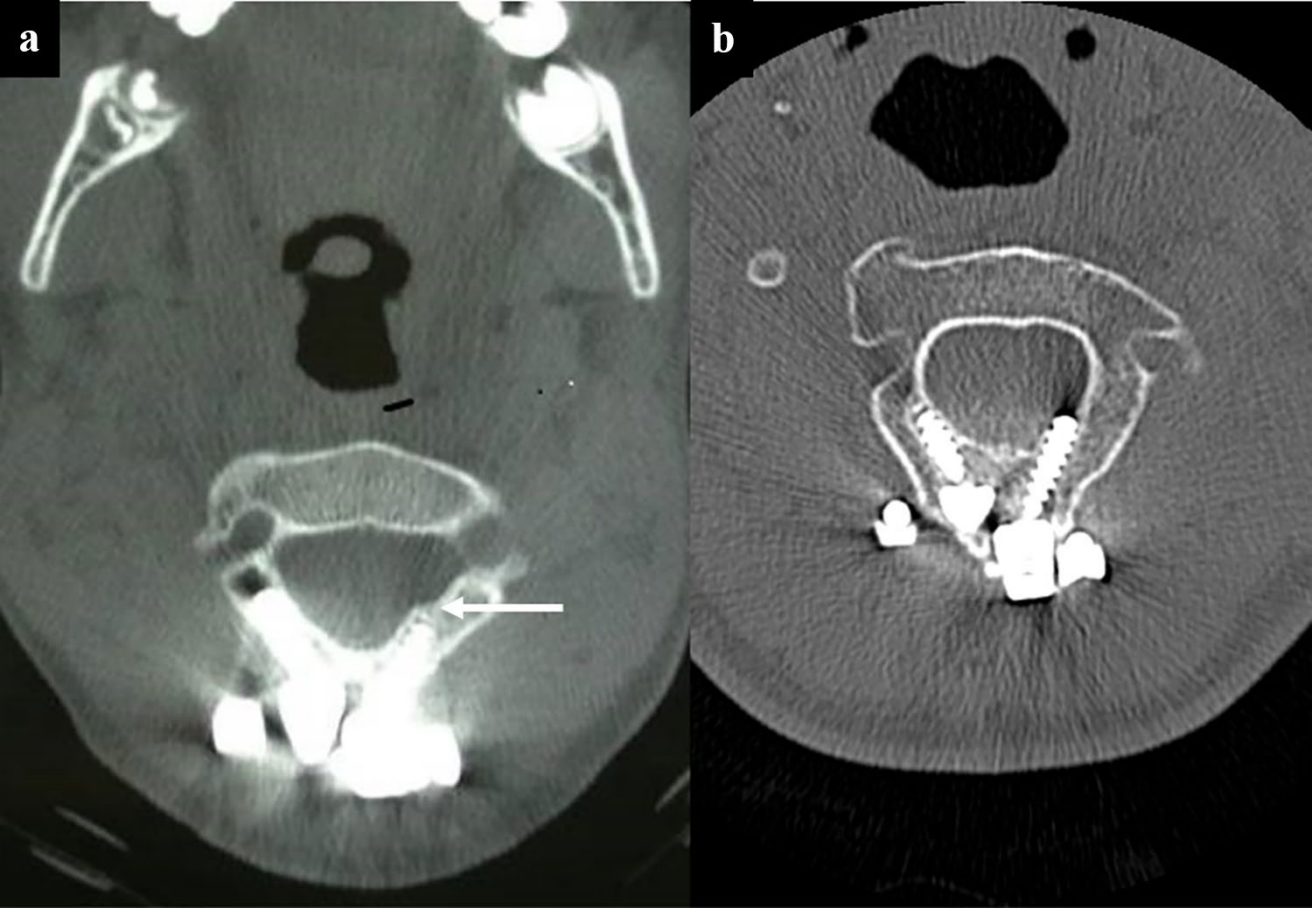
Reduction of surface area for fusion and ventral breach of translaminar screws. a This axial CT scan of the C2 laminae with implanted hardware demonstrates the reduced surface area available for bony fusion due to the presence of the screw heads and the offsets that are necessary for attachment of the translaminar screws to the rod. Of note, this CT scan also demonstrates laminar breech of the left translaminar screw with a fractured cortical shell (white arrow). b This axial CT scan of the same patient taken 7.5 years later to follow-up on the ventral fracture demonstrates that cortical covering of the breech has remodeled and the screw threads protrudes more in the canal without a cortical layer
